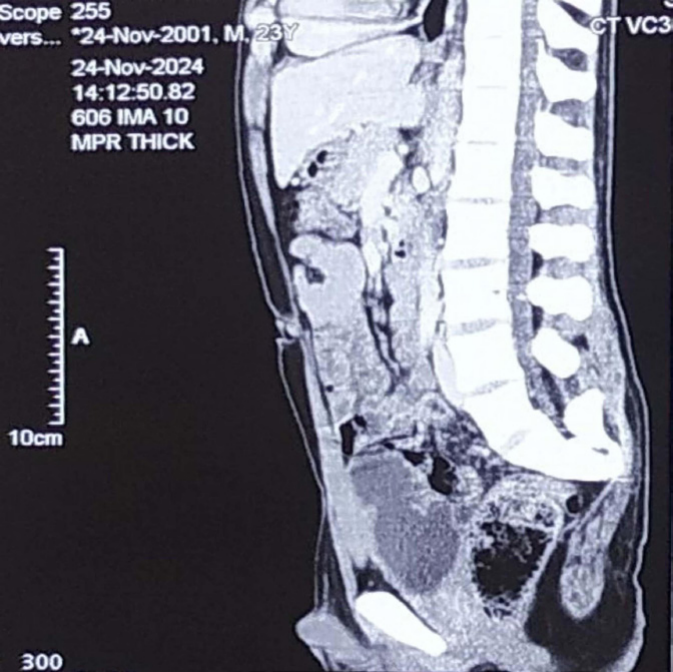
Computed tomography showing sacral agenesis.
